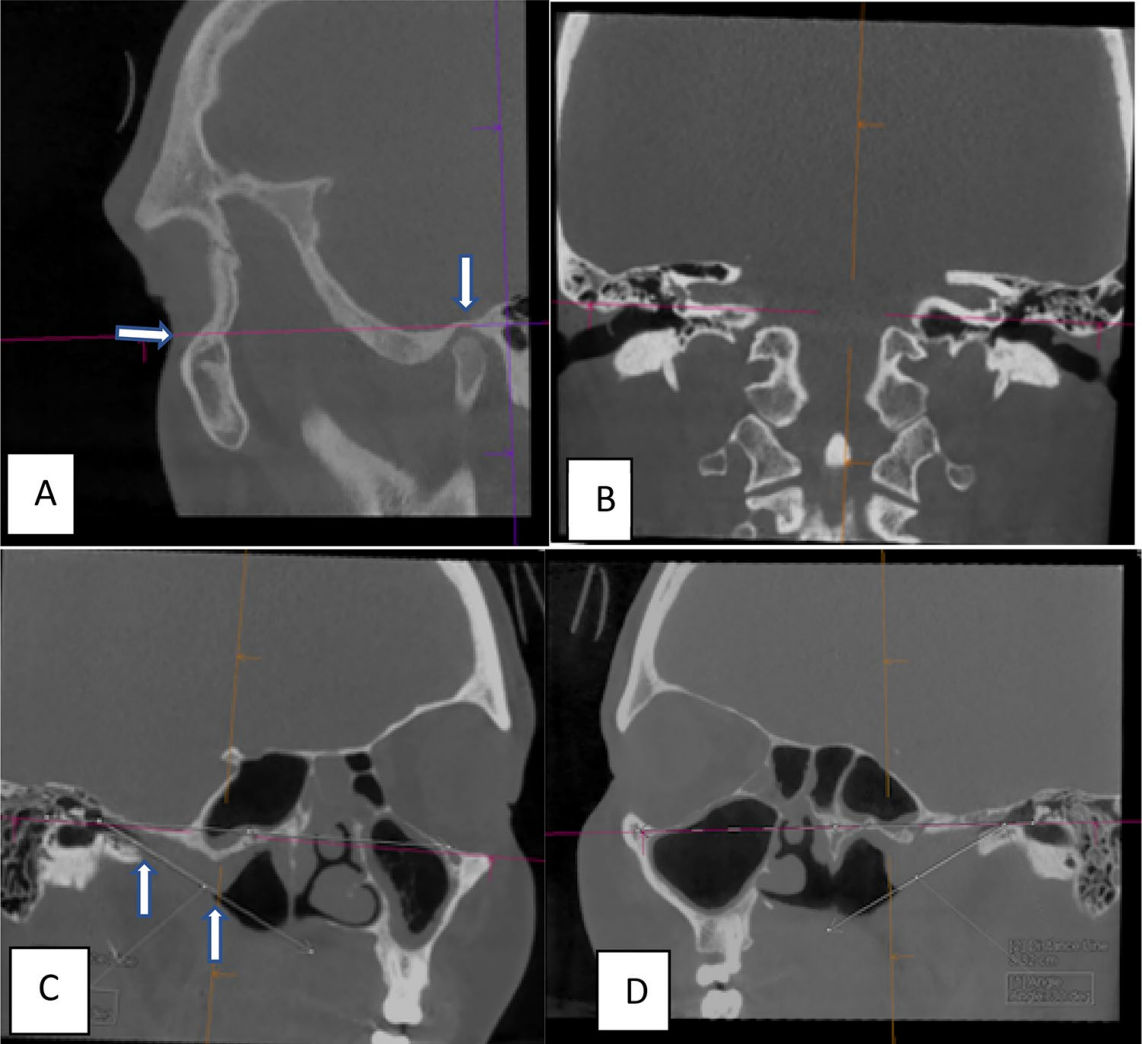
Development of the Ku-Copson plane on MPR analysis of CT PTB scans. A The right infraorbital margin (left arrow), right mandibular fossa (right arrow) and the upper margin of the right EAC are on the same plane. B The plane is adjusted to course through bilateral upper margins of EACs. C The right pharyngeal orifice (right arrow) and tympanic orifice (left arrow) are identified, the distance measured, and ET angle measured with reference to the mandibular fossa plane. D The left ET length and angle were measured
